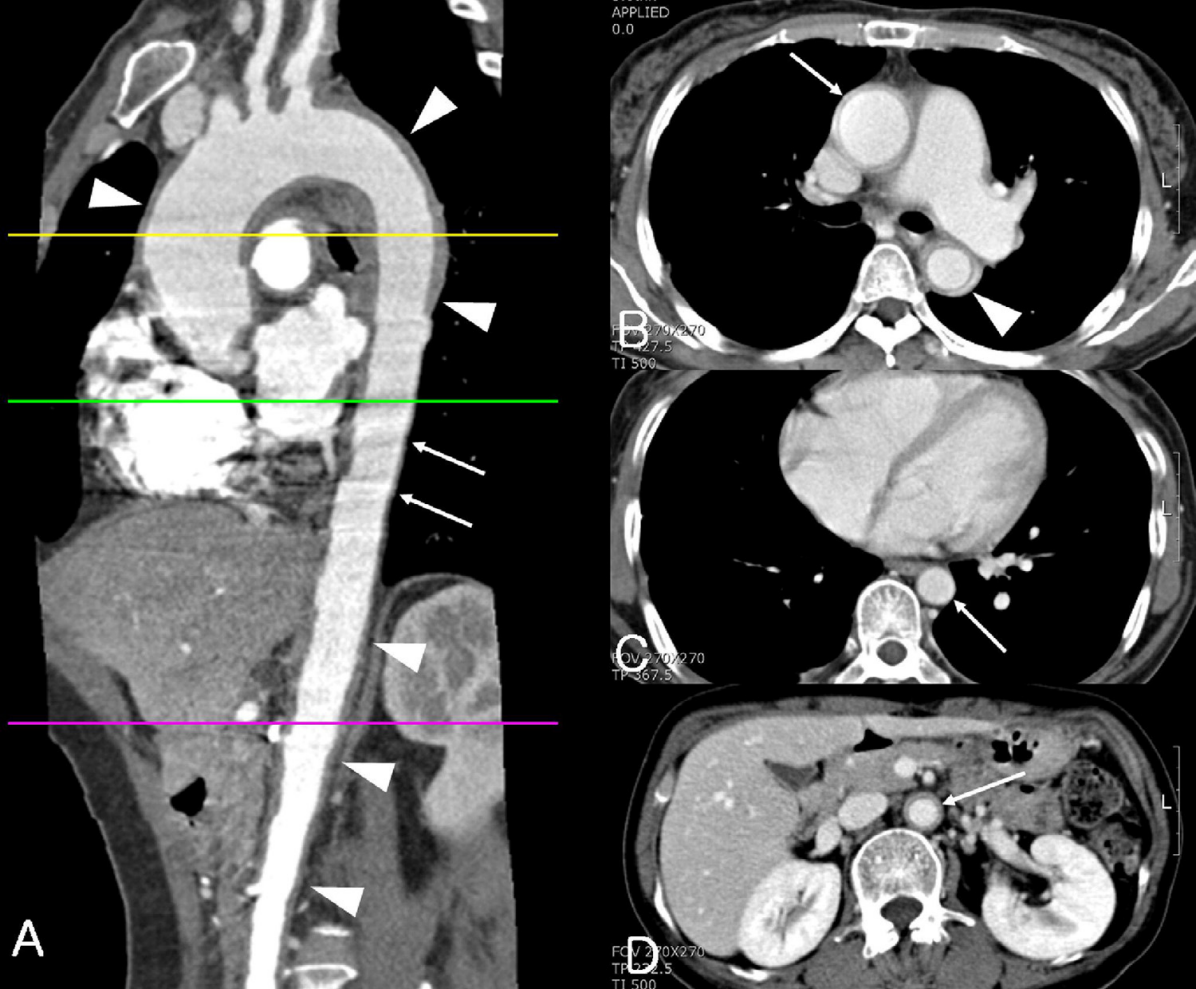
Takayasu arteritis involving aorta with skipped segment in a 50-year-old woman. (A) Multiplanar reformation image shows wall thickening (arrowheads) of ascending thoracic aorta, aortic arch, proximal descending thoracic aorta, and abdominal aorta. No wall thickening of distal descending thoracic aorta is noted. Motion artifact (pulsation artifact) was not observed in the thickened segment such as aortic arch and abdominal aorta due to stiffness of involved aorta and was observed in the distal descending thoracic aorta (arrows) due to pulsation of the noninvolved segment. (B) CT scan at the proximal descending thoracic aorta shows diffuse wall thickening and inner low attenuated ring of ascending (arrow) and descending thoracic aorta (arrowhead). (C) CT scan at the distal descending thoracic aorta (arrow) shows no wall thickening of aorta. (D) CT scan at the abdominal aorta shows diffuse wall thickening and inner low attenuated ring of abdominal aorta (arrow) [15].
